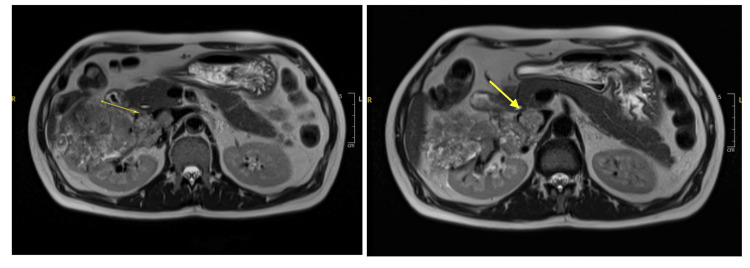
Axial views of MRI kidneys showing a locally advanced right renal mass with tumor thrombus in the right renal vein (left, arrow) and tumor thrombus in the infrahepatic IVC (right, arrow) MRI: magnetic resonance imaging; IVC: inferior vena cava
